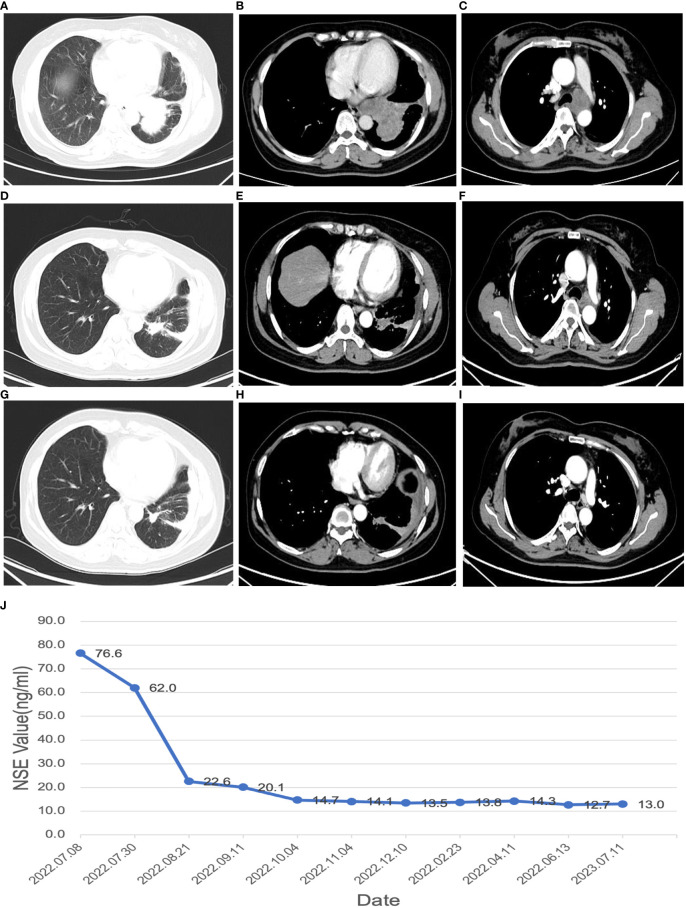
Changes in maximum tumor volume by chest CT scans and changes in NSE value during the disease (A–C), CT images at the time of initial diagnosis; (D–F), CT images after receiving 2 cycles of neoadjuvant therapy; (G–I), CT images after 4 cycles of neoadjuvant therapy; (J), changes in NSE value during the disease.
